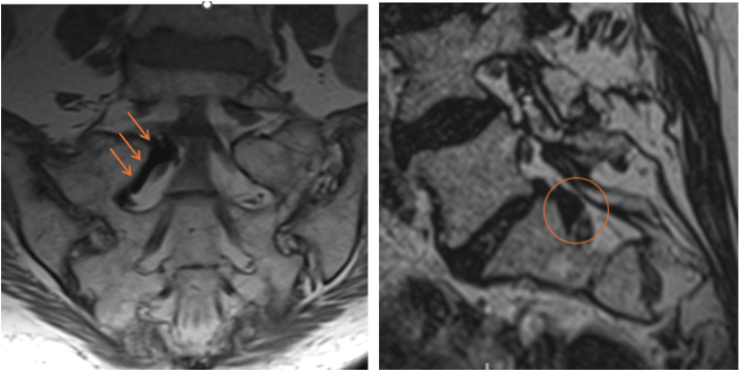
Image 3. Image on left shows *Coronal MRI view demonstrates polymethylmethacrylate cement extravasation with rostral extension toward the L5 level and intracanal extravasation along the expected course of the right S1 nerve root, correlating with the patient's post-procedural radicular symptoms. On the sagittal view, the cement is noted posterior to S1 vertebral body (image on right)*.
